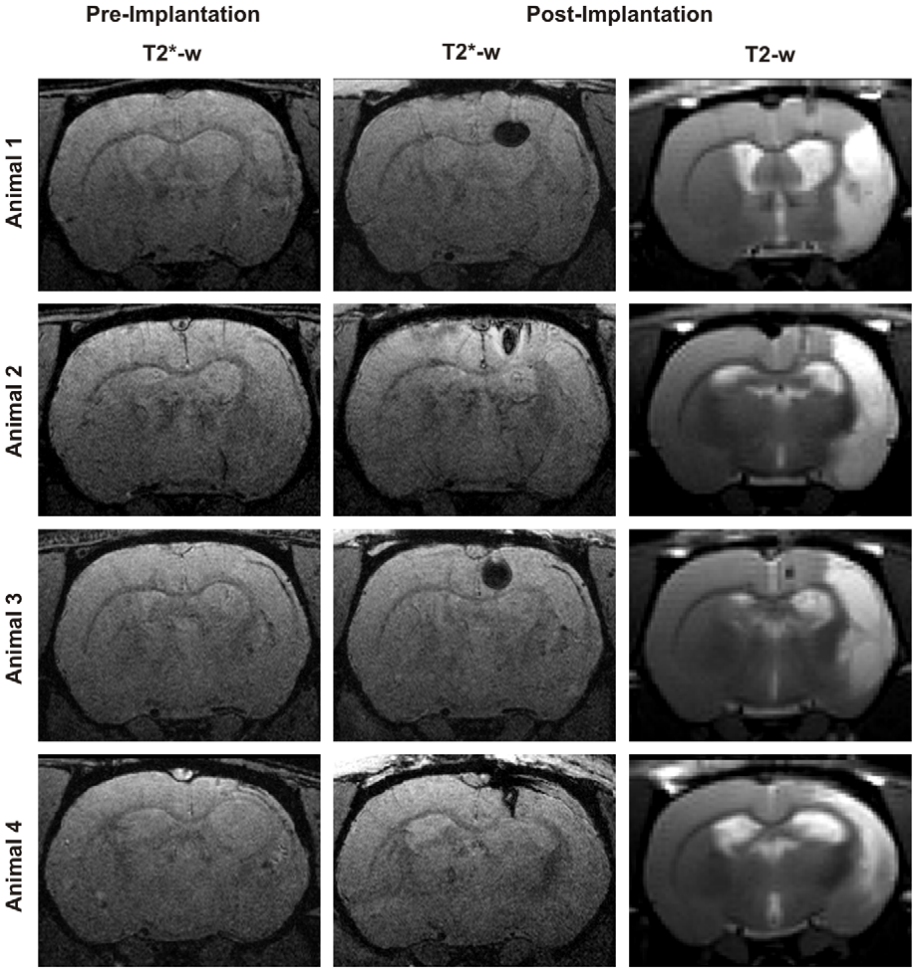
Localization of labeled cells by T2*-weighted MRI. 3D high resolution T2*-weighted MR images, pre- (1st column) and post- (2nd column) implantation of USPIO-labeled stem cells, showing the successful implantation of the cells into the brain parenchyma. Corresponding T2-weighted images (3^rd^ column) confirm that cells were implanted in healthy tissue.
